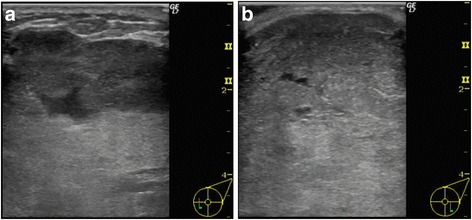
Ultrasonographic findings. a, b Ultrasonographic images showing a large segmental tumor in the left mammary gland
